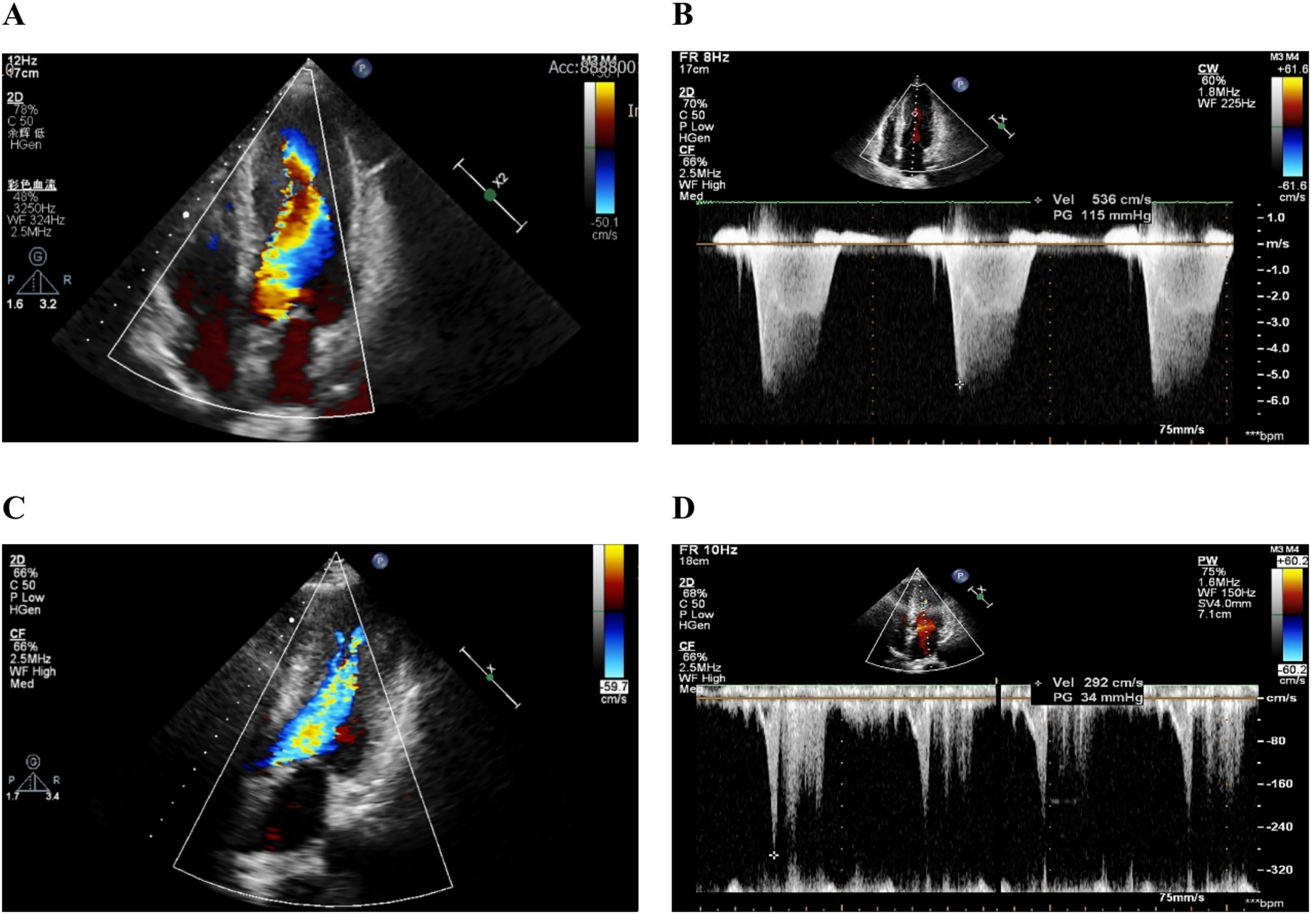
Echocardiographic characteristics at baseline and at 32 weeks follow-up. Color Doppler showing mid-ventricular acceleration before and after mavacamten treatment (A,C); Peak mid-ventricular gradient before and after mavacamten treatment (B,D).
